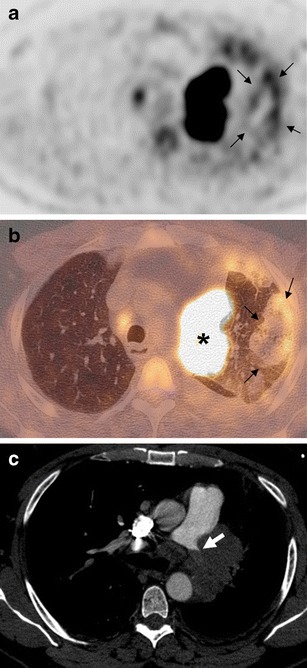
A 53-year-old man referred for small cell lung cancer staging (patient 2). FDG-PET/CT images showed a left upper lobe subpleural consolidation with triangular shape and central lucencies, exhibiting the rim sign (a, axial PET; b, axial fusion, *arrows*). Mediastinal tumor with high FDG uptake is clearly visible (b, *star*). MDCT showed complete arterial obstruction of the left pulmonary artery (c, *arrow*)
